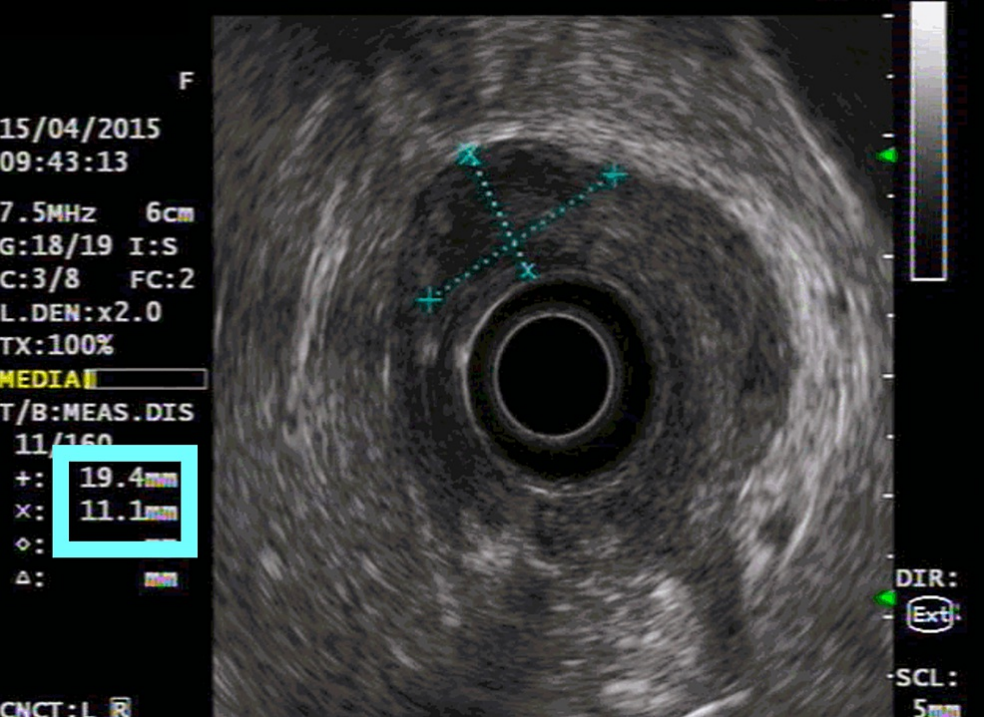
Endoscopic ultrasound at diagnosis We performed an endoscopic ultrasound (EUS) for the locoregional disease staging, with an estimated depth of invasion of 19.4 mm (blue box)
